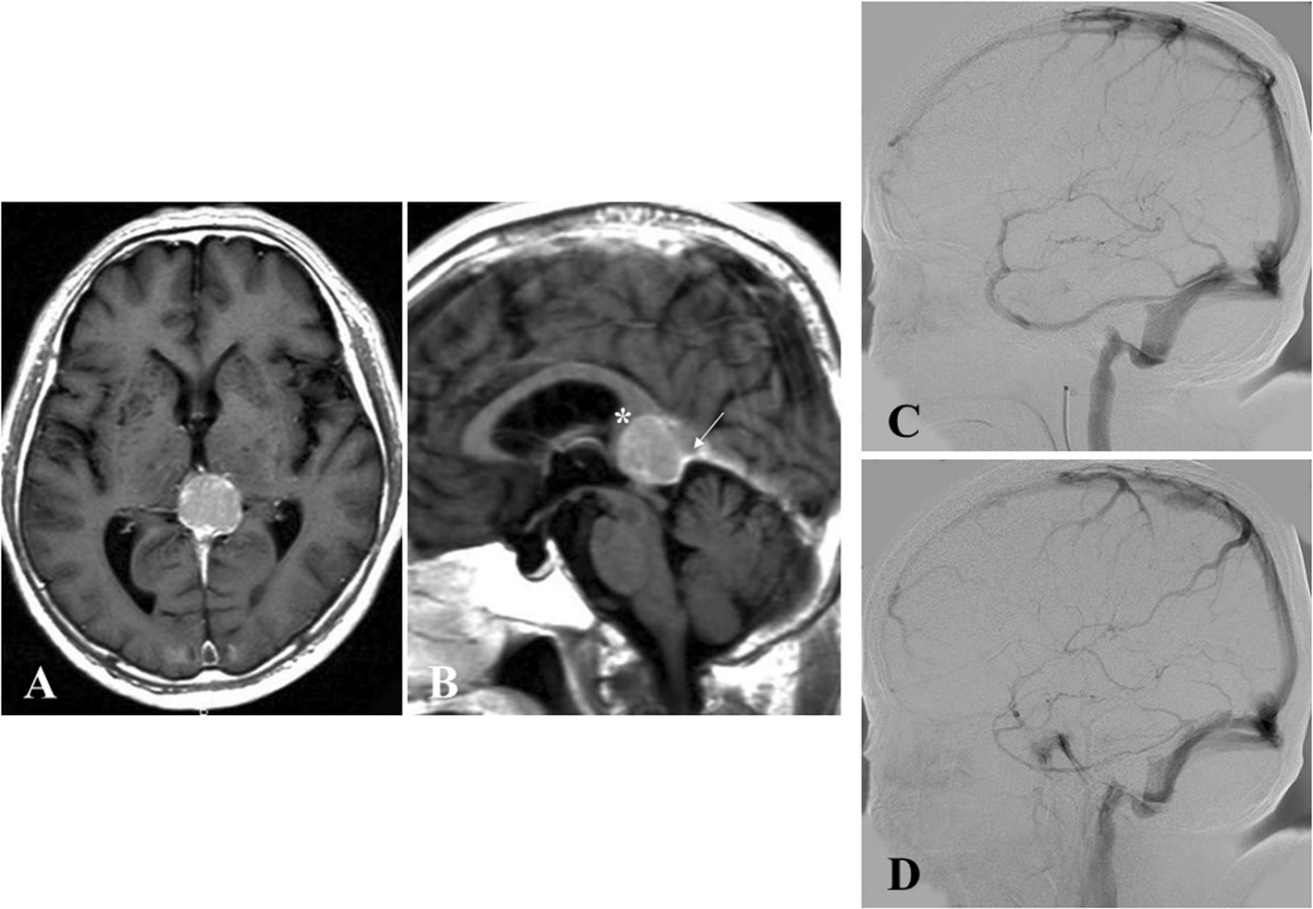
Radiological imaging of the tumor in the pineal region in Case 2. a, b Gadolinium-enhanced T1-weighted imaging in Case 2 shows a well-demarcated tumor in the quadrigeminal cistern, compressing the pineal gland and splenium of the corpus callosum (*white asterisk*) anteriorly (a, axial view, b sagittal view). The vein of Galen (*white arrow*) is displaced downward. c, d Preoperative ICAG shows occlusion of the vein of Galen and deep venous flow draining through the collateral venous channel into the transverse sinus (c right venous phase, d left venous phase)
